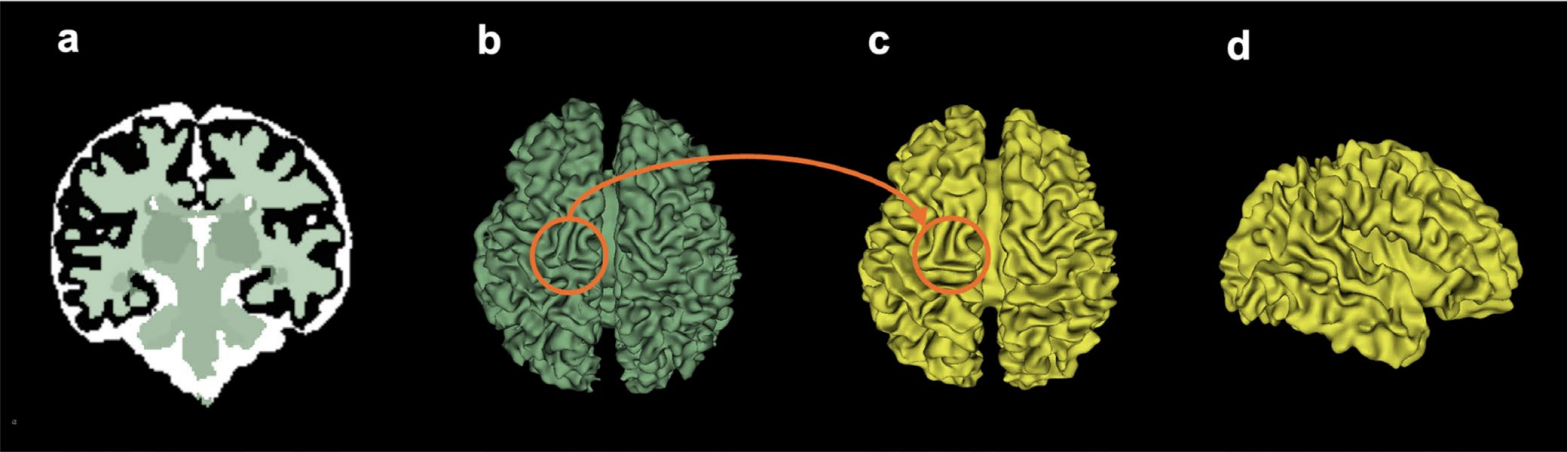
Reconstruction of the white matter surface from the second time point (later‐stage scan). (a) Automatic segmentation of the original MRI image at 38 gestational weeks, (b) The extracted white matter surface without manual modification, (c, d) Axial and sagittal views of the white matter surface following manual modification, smoothing, and remeshing.
